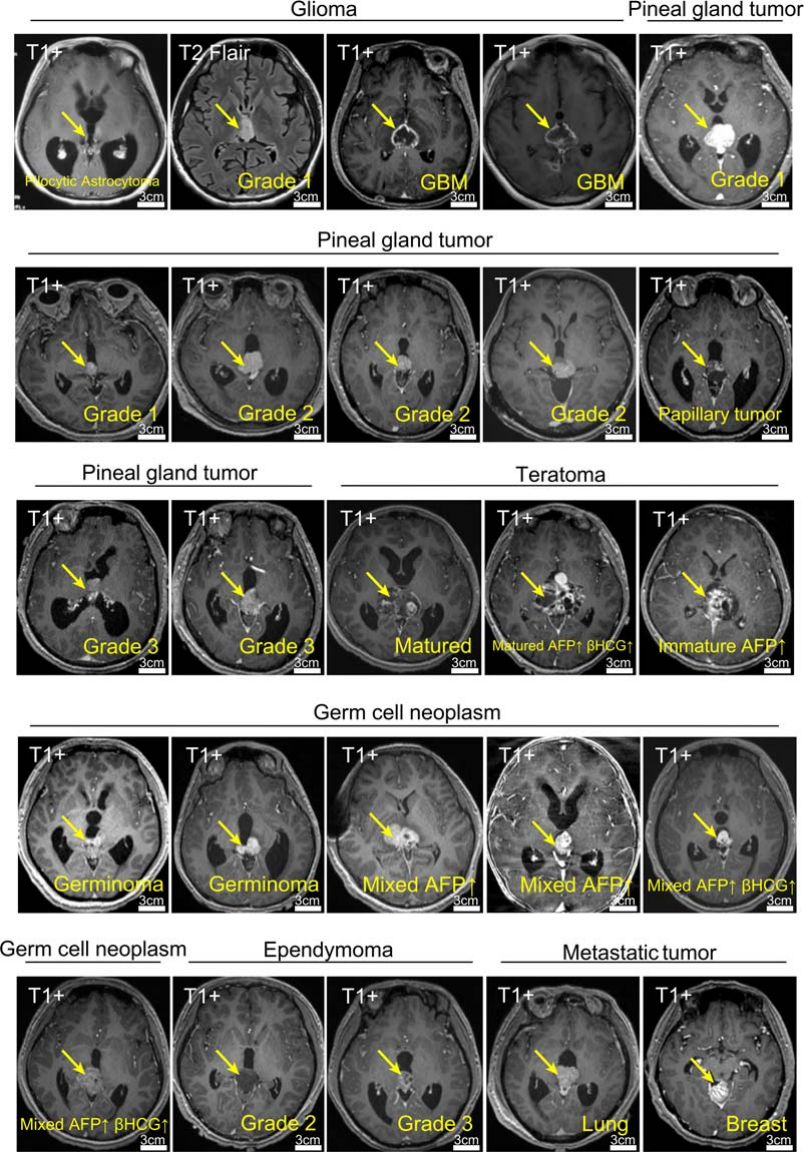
The category of 25 pineal region tumors with annotation of pathology, grade, and some laboratory information, and tumor marked with arrowheads. The scale bars are shown in the MR images. AFP, α-fetoproteinand; β-HCG, β-human chorionic gonadotropin.
